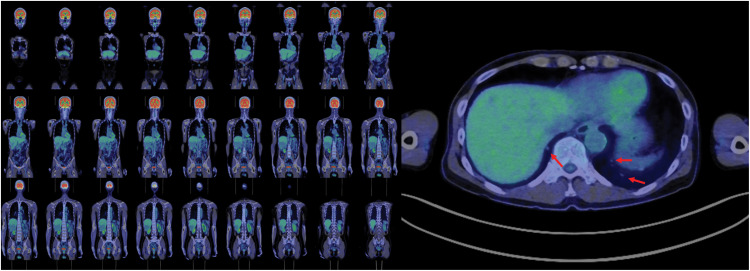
PET-CT images. Neither the pulmonary nodules nor the liver tumors showed abnormal fluorodeoxyglucose uptake (lung nodules are indicated by red arrows).
